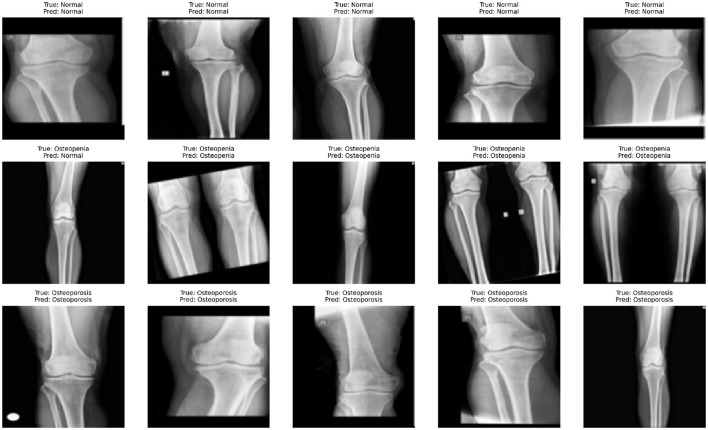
Classification results of the TSFGDTDBN model for Multi-Class Knee Osteoporosis X-ray Dataset.
